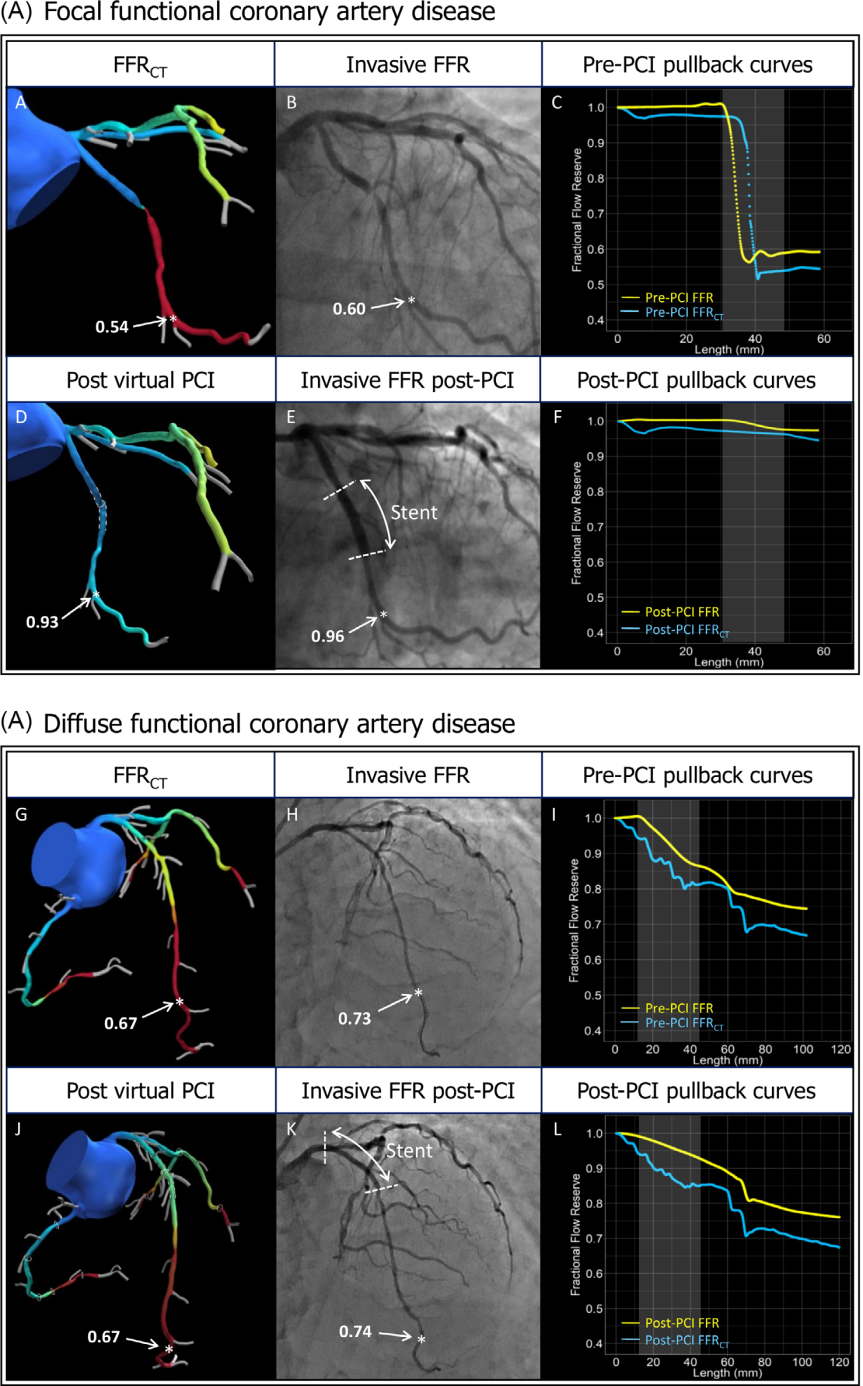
Case example of the application of FFR_CT_Planner incases with focal and diffuse functional coronary artery disease. (A) Focal functional coronary artery disease. Panel A shows the FFR_CT_ model showing a focal, hemodynamic significant lesion in the Circumflex coronary artery. Panel B shows invasive angiography confirming an angiographic focal lesion. The position of the pressure wire sensor is denoted by a white star. Panel C shows the FFR_CT_ and invasive FFR pullback curves. Panel D shows the remodeled geometry (white dashed lines) and presents the results of the blinded luminal remodeling using the FFR_CT_ Planner. Panel E shows the location of distal invasive FFR assessment post‐PCI (white star) matched with the FFR_CT_ model. The FFR_CT_ Planner predicted a FFR_CT_ value of 0.93 at the same position (white star) where the invasive FFR post‐PCI recorded 0.96. Panel F shows the corresponding post‐PCI pullback curves derived from FFR_CT_ and invasive FFR (blue and yellow lines, respectively). (B) Diffuse functional coronary artery disease. Panel G shows a patient‐specific FFR_CT_ model with diffuse pressure loss along the LAD and distal FFR_CT_ value of 0.67. Panel H shows invasive coronary angiography with distal invasive FFR value of 0.73 (white stars). Panel I shows the FFR_CT_ and FFR pullback curves pre‐PCI. Panel J shows the remodeled segment in the FFR_CT_ model (white dashed lines) predicting a FFR of 0.67. Panel K shows the location of the invasive FFR measurement of 0.74 (white star). Panel L shows the post‐PCI pullback curves derived from FFR_CT_ and invasive FFR (blue and yellow lines, respectively). FFR_CT_ Fractional flow reserve derived from CT. FFR, fractional flow reserve; LAD, left anterior descending artery; PCI, percutaneous coronary intervention
